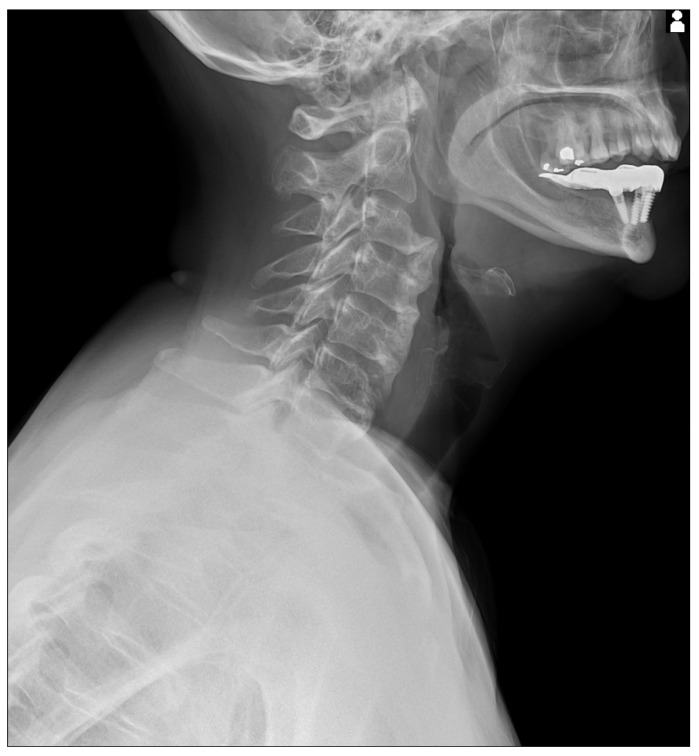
Static X-Ray acquisition acquired with Konica Minolta (AeroDR TX, Konica Minolta Inc., Tokyo, Japan) with a static setting. The cervical X-Ray shows florid, flowing ossification along the anterior or right aspects of five contiguous vertebrae, from C3 to C7 [1,2,3,4,5,6]. The intervertebral disk spaces are preserved.
